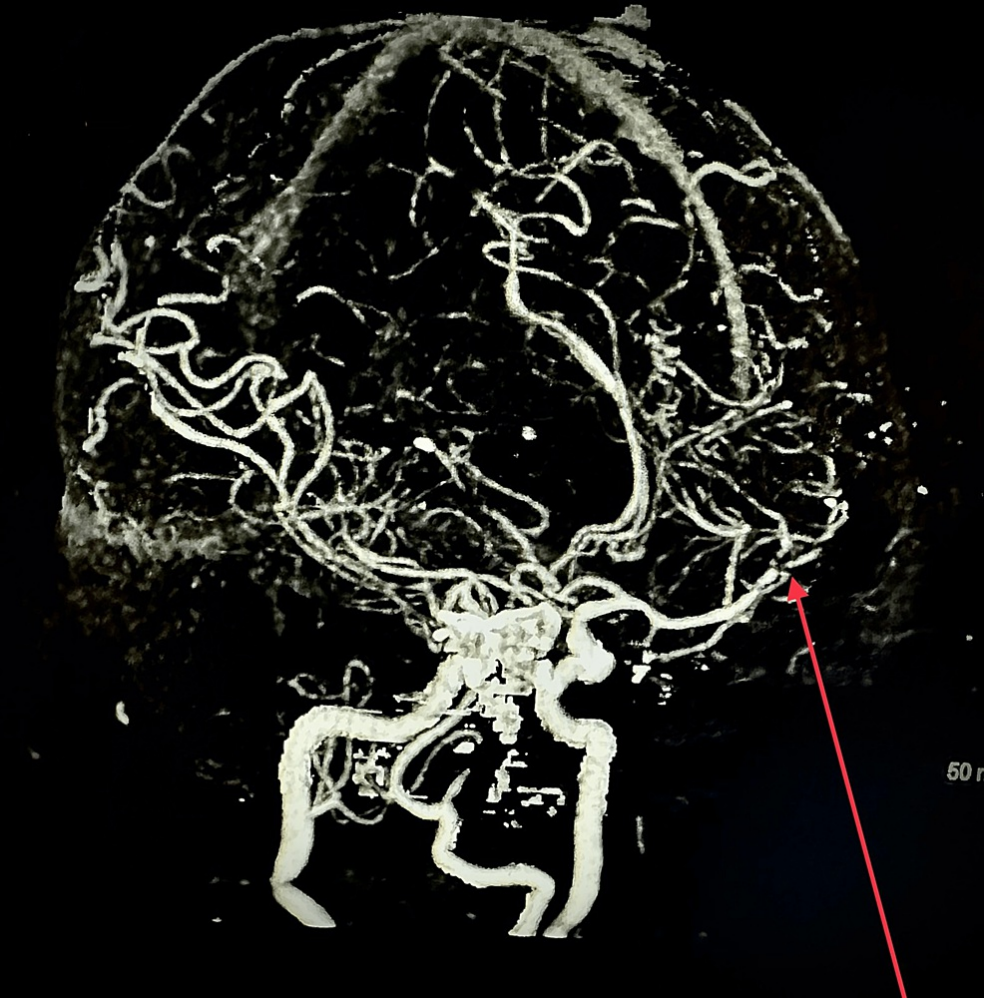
CT angiogram of the head and neck with and without contrast showing high-grade proximal stenosis of the right M3 segment. CT, computed tomography
